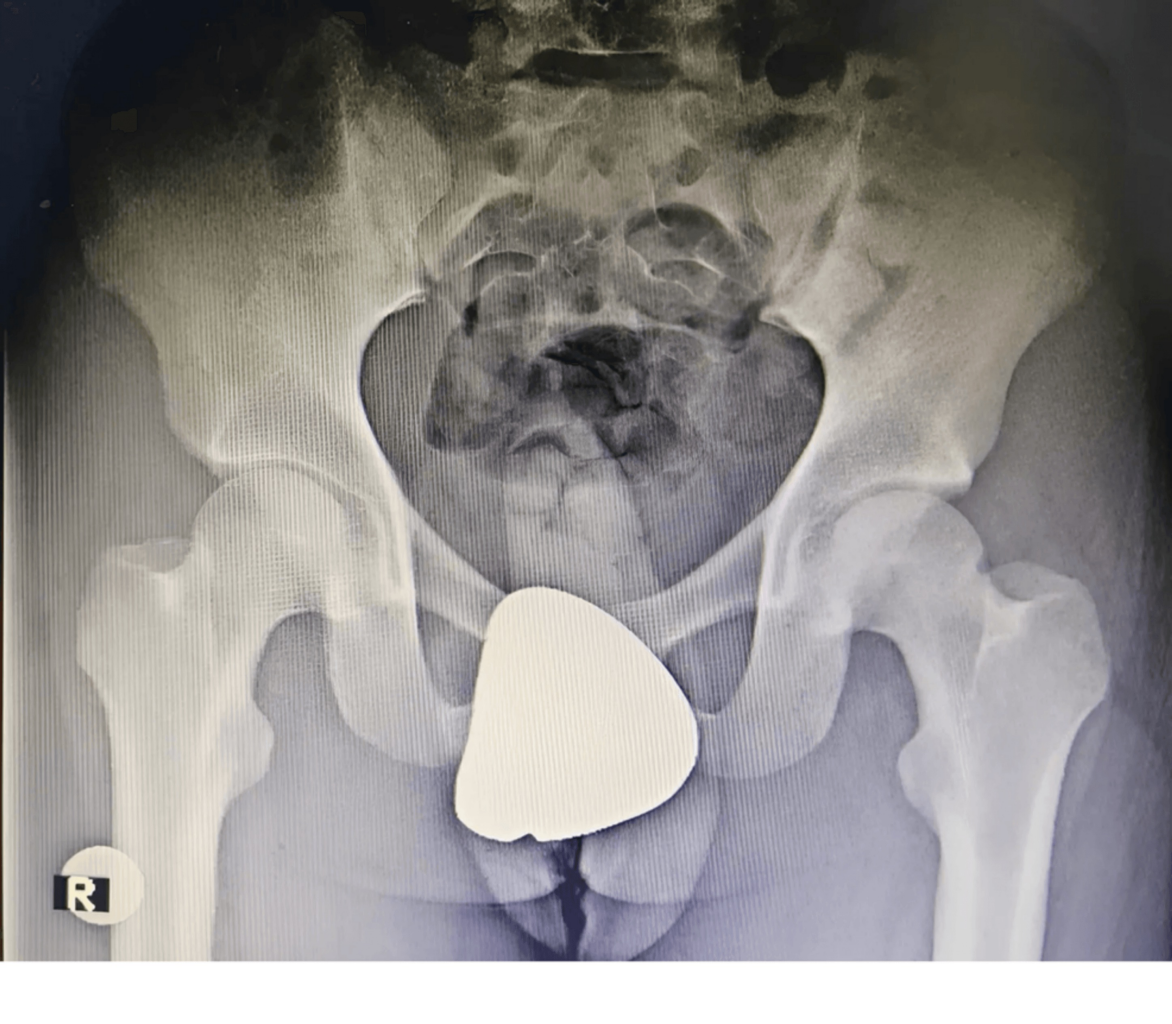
Anteroposterior radiograph next day after close reduction shows relocation of the femoral head.
